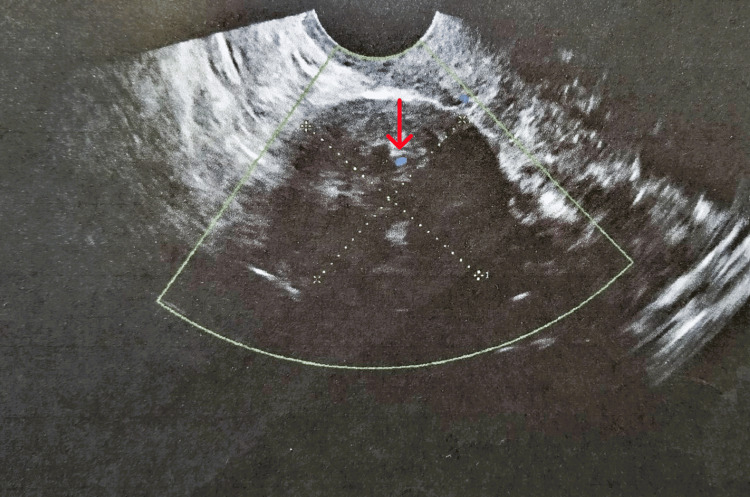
Transvaginal ultrasound and Doppler ultrasound imaging of an ovarian fibroma (our case) A solid hypoechoic mass with clear margins and minimal Doppler flow signals (red arrow) are typical ultrasound features of ovarian fibroma
